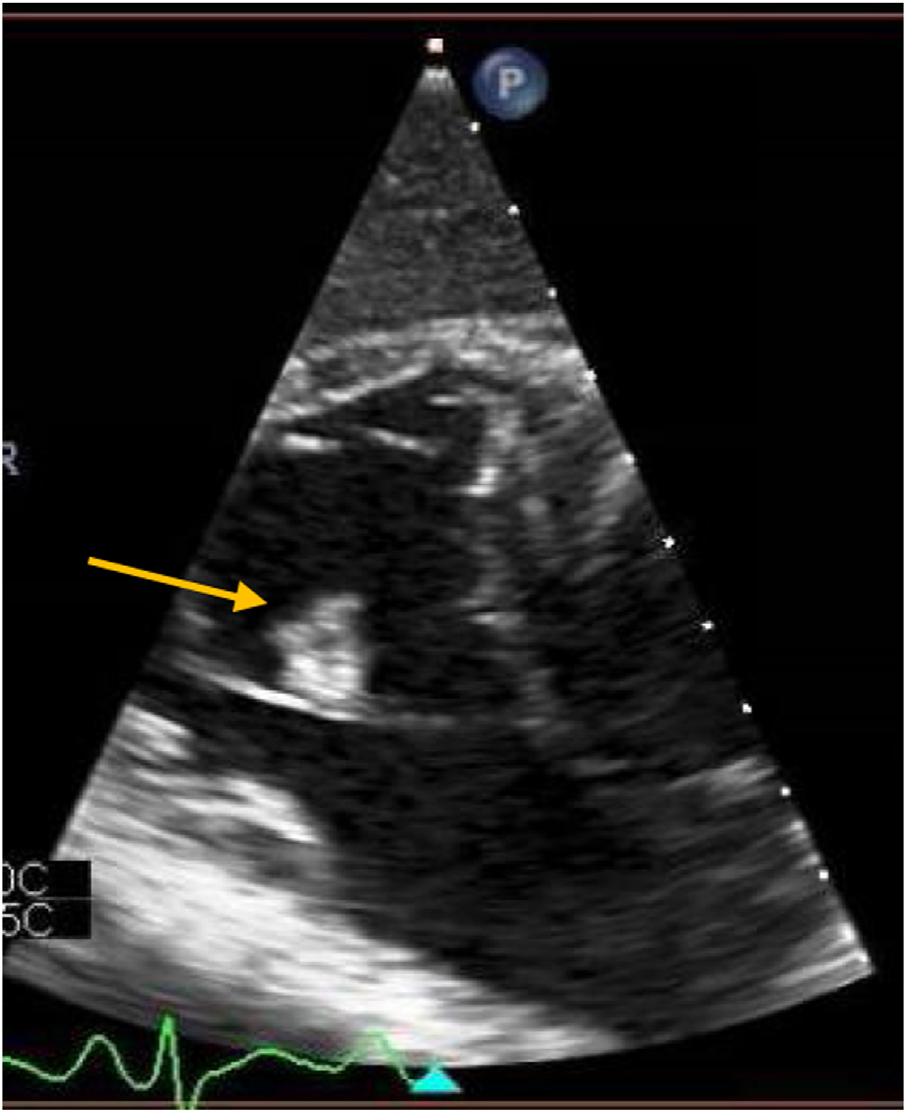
Transesophageal Echocardiography showing rectangular shaped vegetation attached to the entire length of anterior tricuspid leaflet, measuring 1.8 * 1.5 cm.
